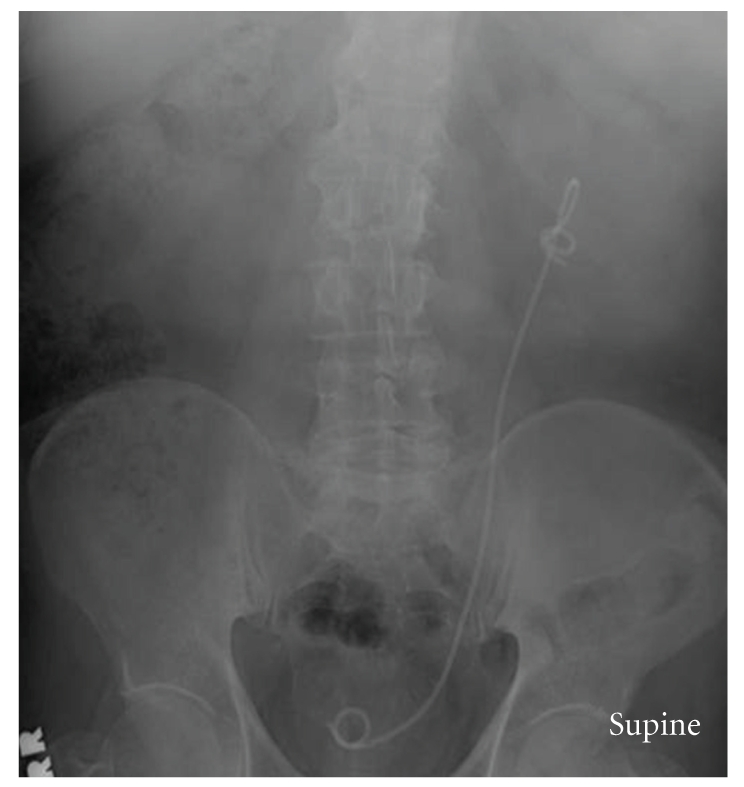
A plain X-Ray film shows the coiled proximal part of the JJ stent with formation of an incomplete knot.
